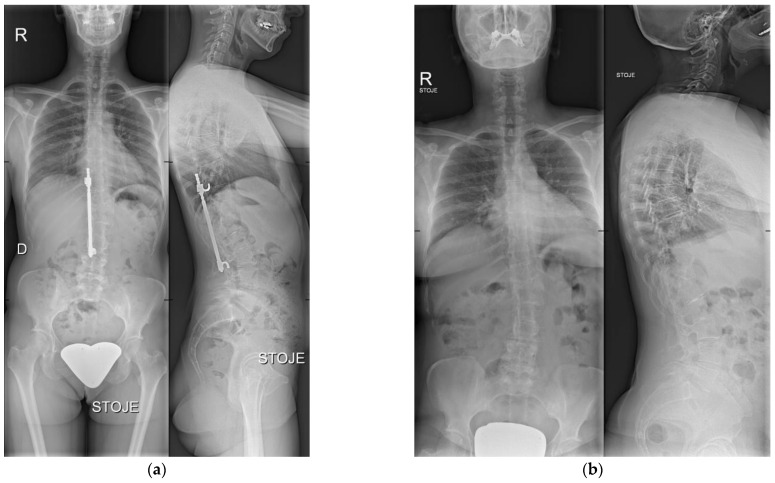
(a) X-ray taken before HR instrumentation removal. Anteroposterior (left) and lateral (right) view. (b) Most recent X-ray after HR instrumentation removal. Anteroposterior (left) and lateral (right) view.
